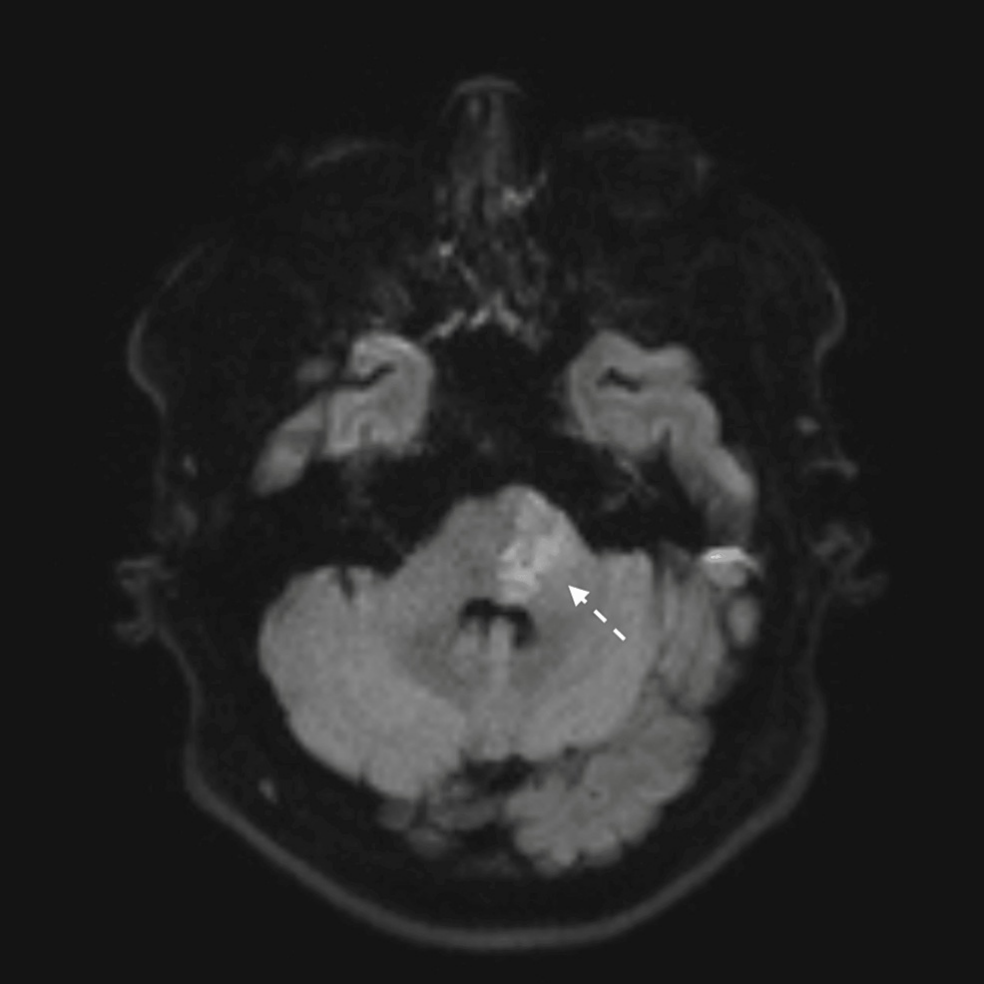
MRI brain DWI view showing left pontine diffusion restriction.
